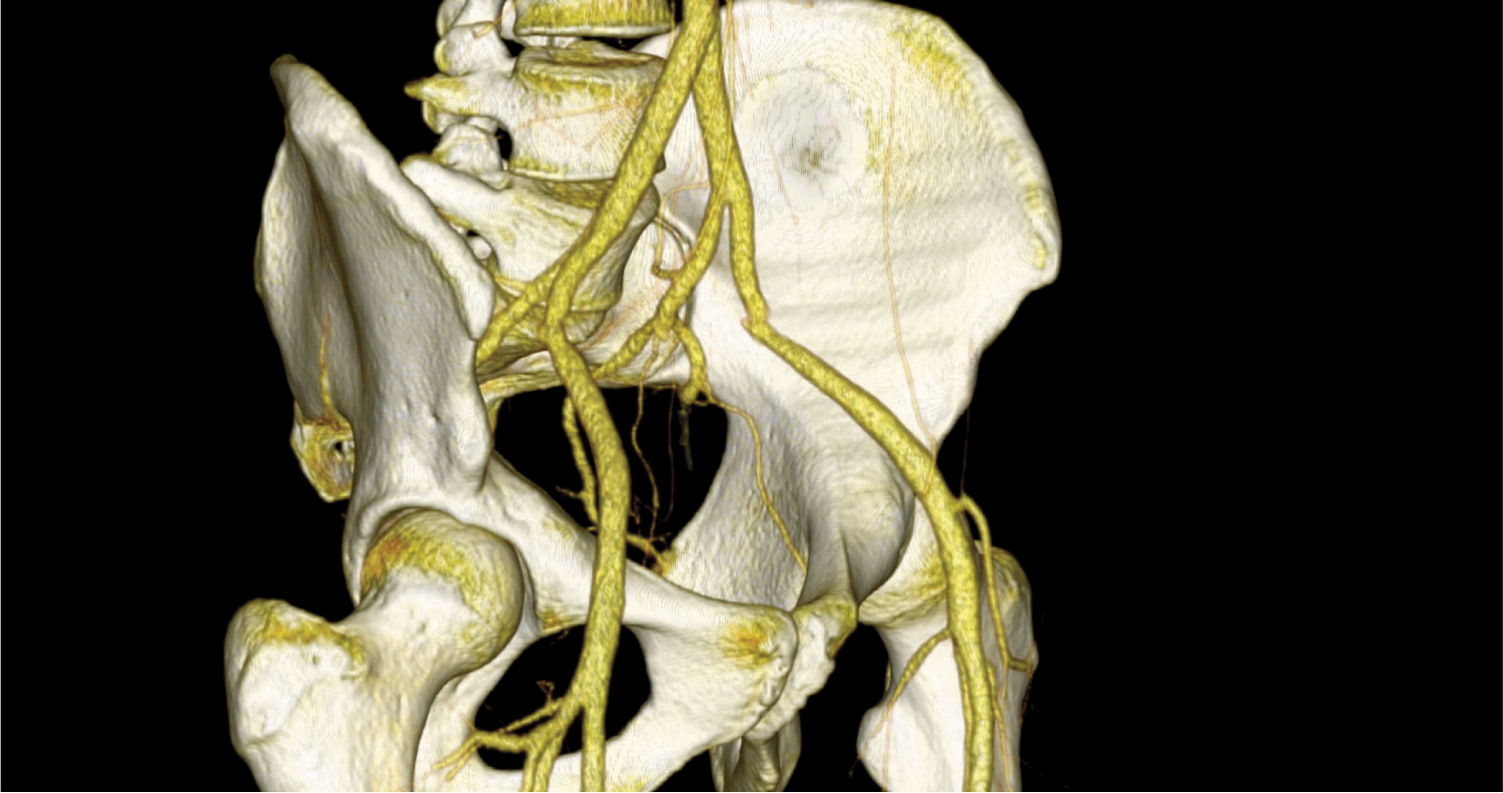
A 3-dimensional reconstruction of the patient's computed tomographic angiogram of the abdominal aorta and common iliac arteries, revealing the stenosis and kink in the proximal half of the left external iliac artery (LEA). We can observe two atypical branches of the LEA; one climbing towards the ilium and the other passing anterior to the left femoral head. Poststenotic dilation is noted. (The patient agreed to the publishing of his radiological images.)
